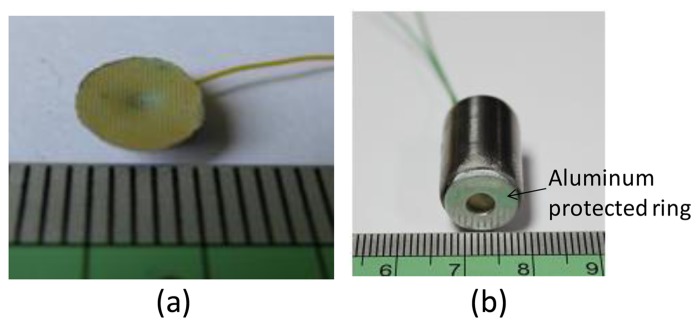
(a) Finished ultrasonic transducer with an acoustic impedance gradient matching layer; (b) The fabricated transducer packaged in an aluminum tube.
